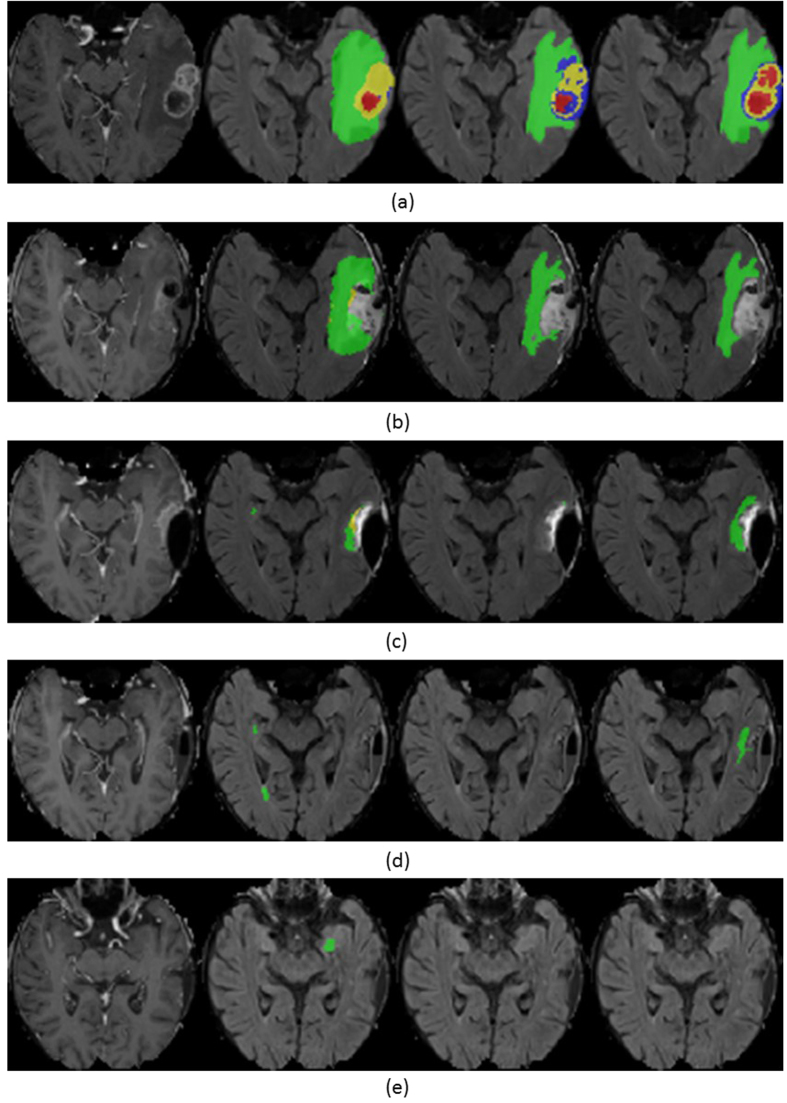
Segmentation of necrosis (red), edema (green), enhancing tumor (yellow) and non-enhancing tumor (blue) in patient “d”. From top to bottom, consecutive time points are shown. From left to right: *T*_1_ post-contrast-weighted image, FLAIR-weighted image overlayed with segmentation generated by BraTumIA, segmentation generated by Rater-1 and segmentation generated by Rater-2.
